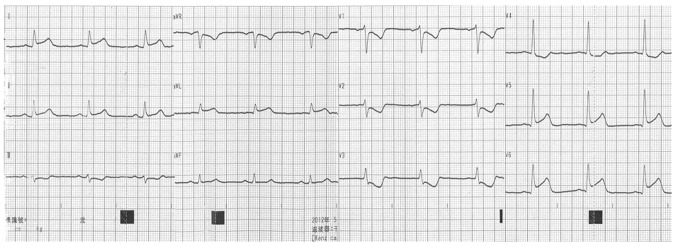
Echocardiogram of case 1 showing a significant depression in the V1-4 ST segment, inversion of the T wave and an elevation of the I, AVL and V6 ST segments.
